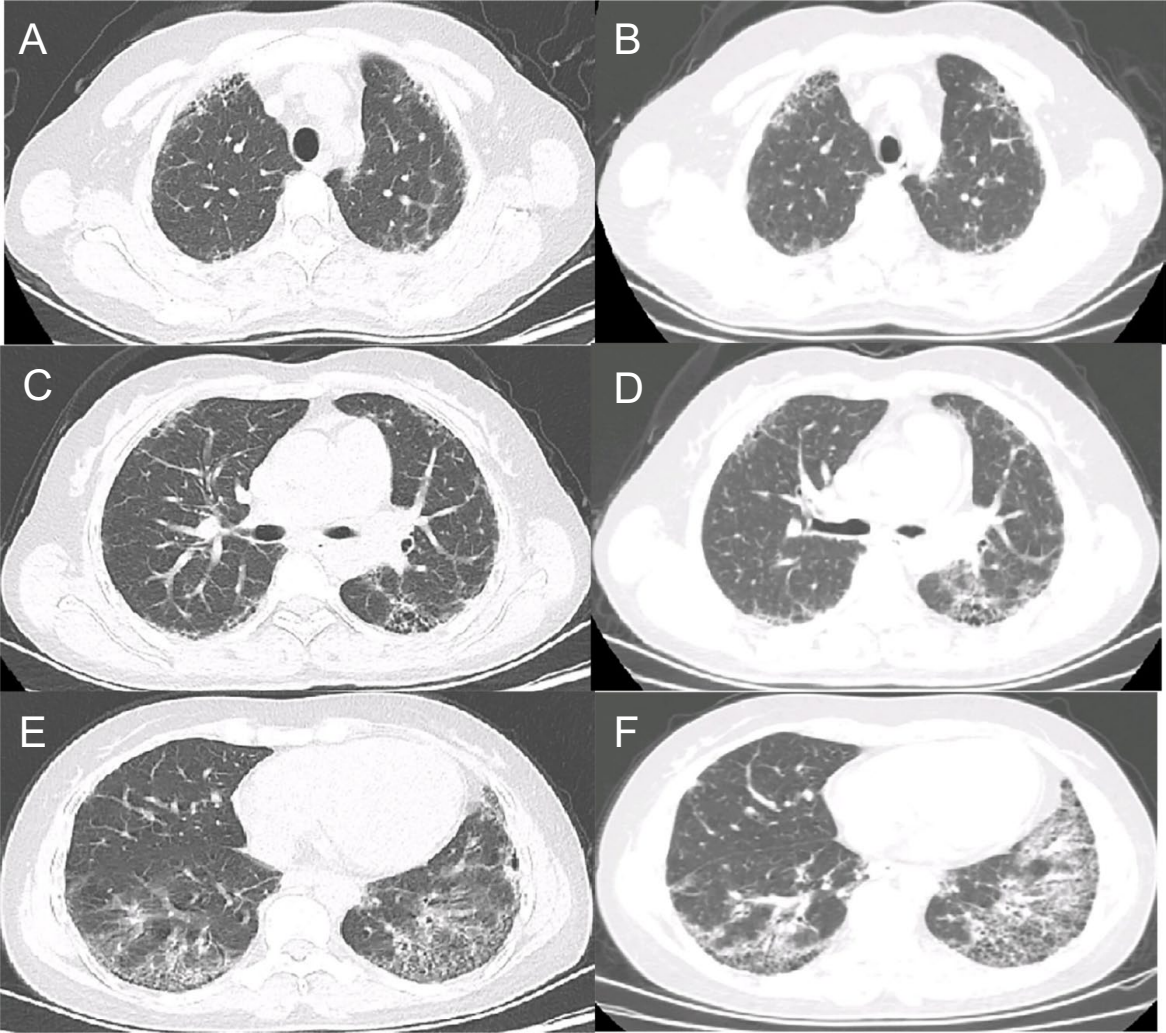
Progressive pulmonary fibrosis due to probable usual interstitial pneumonia. A 32-year-old female with anti-Jo-1 antibody-positive dermatomyositis/interstitial lung disease. A, C, E High-resolution computed tomography (HRCT) images showing moderate honeycombing and reticular abnormalities in the subpleural and basal regions. B, D, F HRCT images showing a substantial increase in the extent of reticular opacities and ground-glass opacities associated with traction bronchiectasis and increased lobar volume loss at the 1-year follow-up
